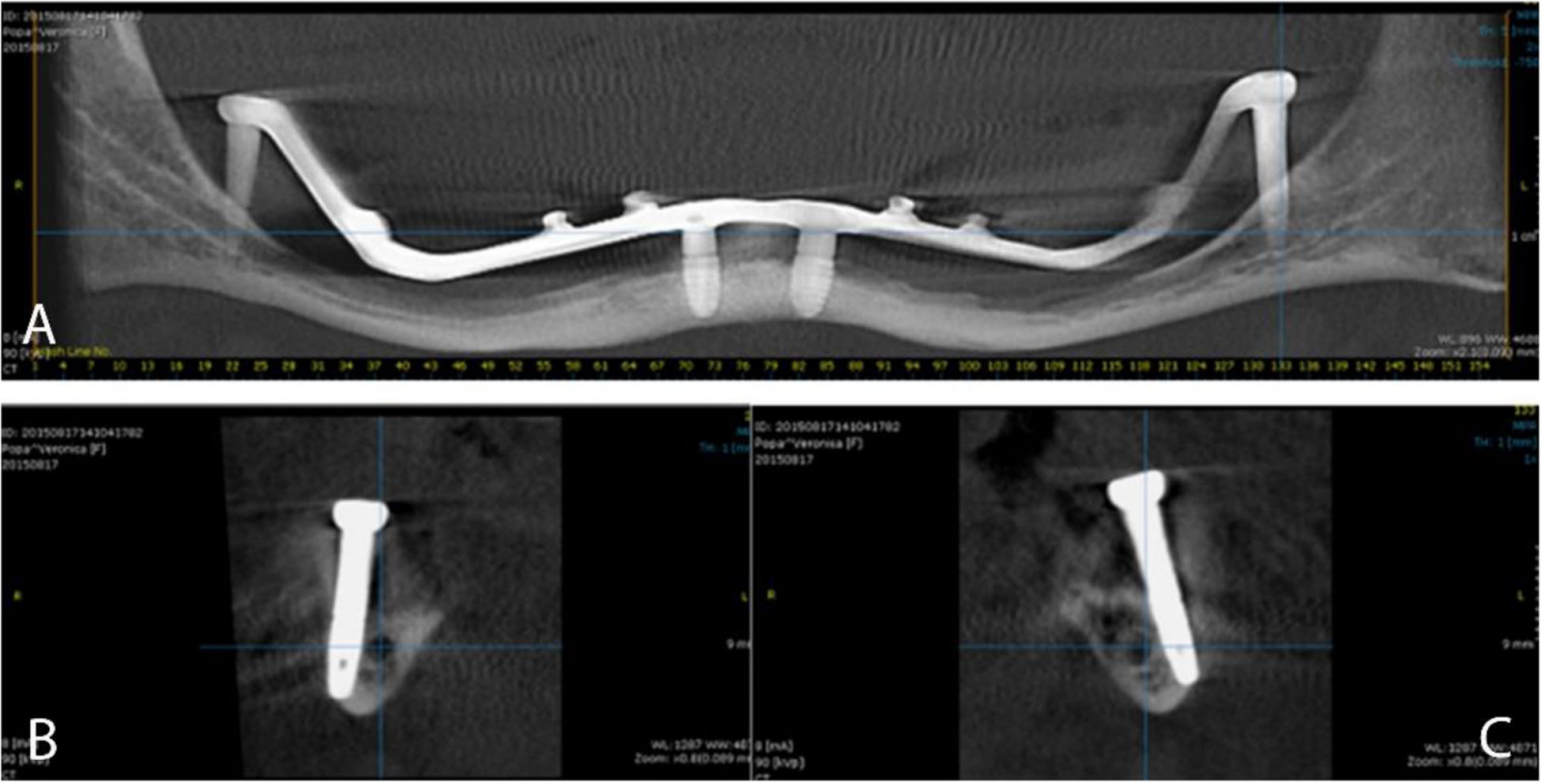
CBCT at 14 months follow-up. A Coronal view of the mandible with implants inserted and attached bar structure; B Sagital right and C Sagital left sections. The residual bone integrity and the absence of resorbtion could be observed in all sections
